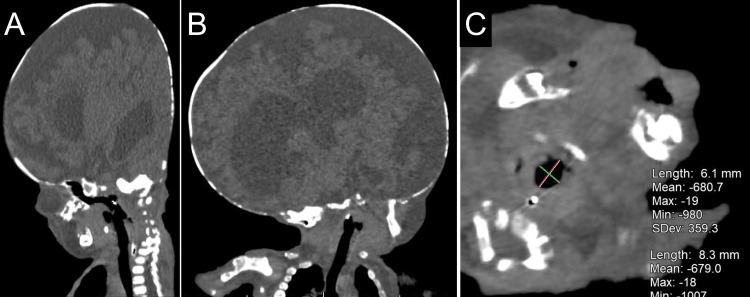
Computed tomography findings of the patient (A, B) No airway stenosis is observed. (C) The diameter of the narrowest part of the trachea is 6.1 x 8.3 mm, which is large relative to the body size.
